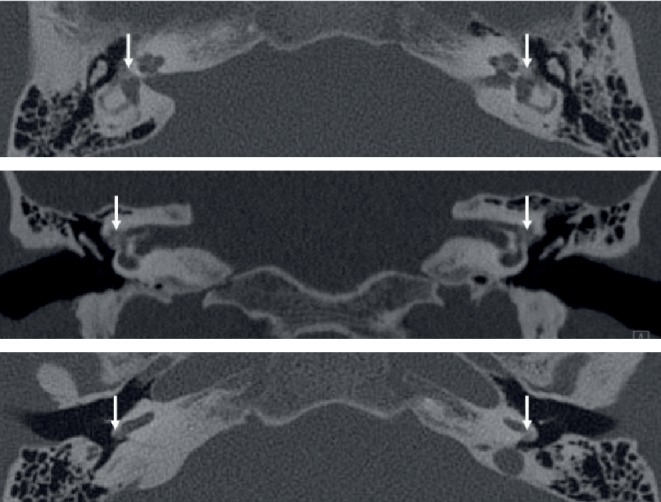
High resolution CT Temporal axial (top and bottom row) and coronal images (middle row) in another case showing ante-fenestral otosclerosis manifesting as ill-defined lucencies (arrows, top and middle row). Note even involvement of round windows (arrows, bottom row).
